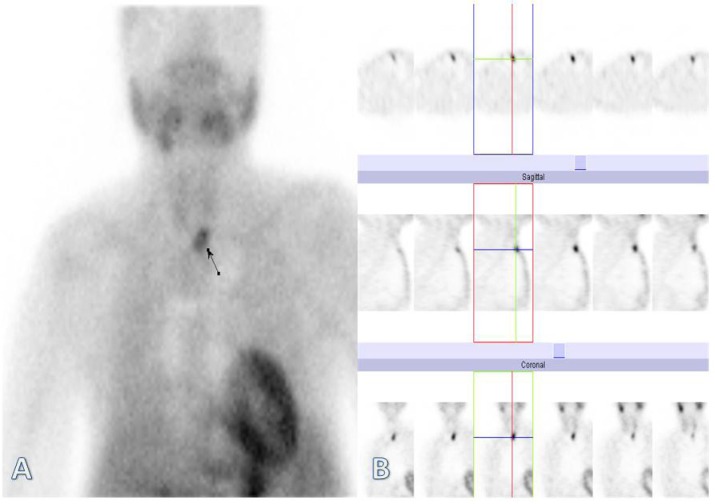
Tc-99m sestamibi scintigraphy of the parathyroid. The delayed phase images on planar (A) and SPECT views (B), showed tracer washout from the neck region with a focal area of retained activity in the left lower part of the neck inferior to the thyroid
